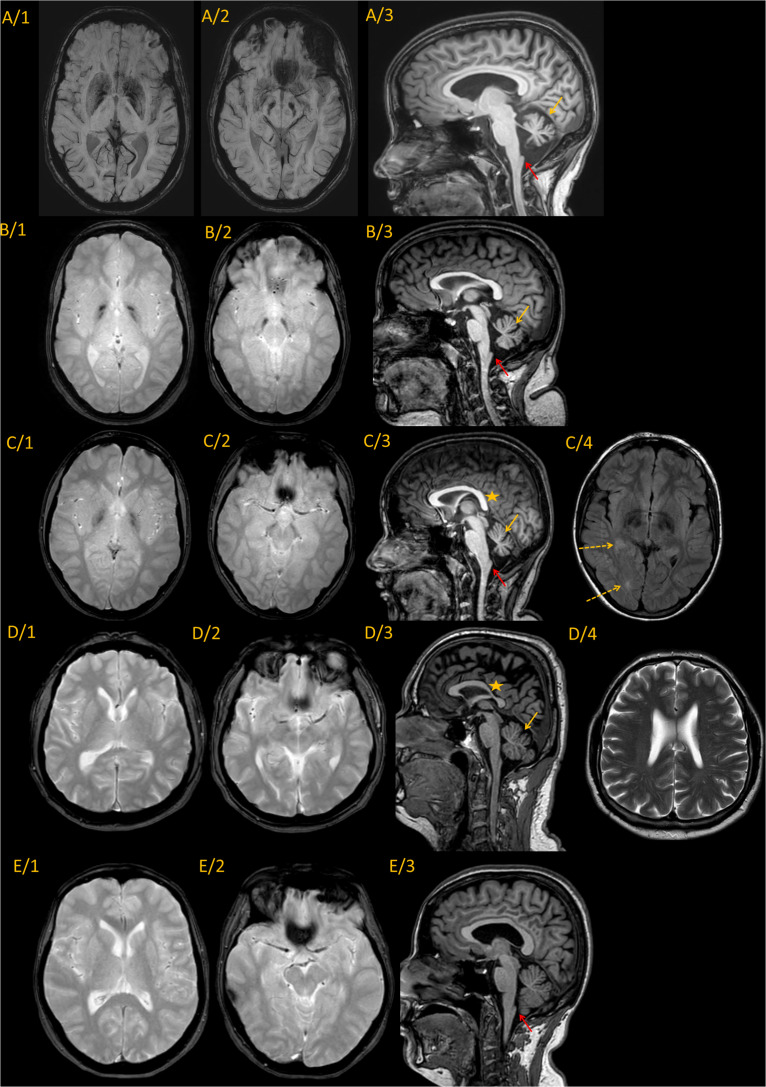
Representative MRI imaging pictures of the family. Every line represents images from a single individual. (A) IV/2; (B) IV/3; (C) IV/4; (D) III/3; and (E) III/4 Column 1–2. Axial iron sensitive sequences (SWI in line A, and T2* in the remaining) through the basal ganglia and midbrain. Column 3: Sagittal midline T1 images. Findings are summarized in the associated table. Every patient diagnosed with PLAN (A–C) showed iron accumulation in the globi pallidi internus and substantiae nigrae, while the carrier parents did not show iron accumulation. Cerebellar atrophy was present in all the three affected children [(A–C), yellow arrows], and was also present at the father [(D/3), yellow arrow]. The characteristic vertical splenium corpus callosum was present at patient IV/4 [(C/3), yellow star], however, also at the father corpus callosum was atypical [markedly thin isthmus in an adult; (D/3) yellow star]. The apparent claval hypertrophy sign was present at all three affected children [(A–C), red arrow], and was identifiable at also the carrier mother [(E/3), red arrow]. Besides, patent IV/4 also had white matter lesions identifiable on T2 images [(C/4), dotted arrows]. At the father enlarged vascular spaces were detected (D/4). In summary, it seems, that besides the characteristic PLAN-associated signs at the affected children, both parents showed mild abnormalities, which might be related to the PLA2G6 carrier state, without pathological iron accumulation.
